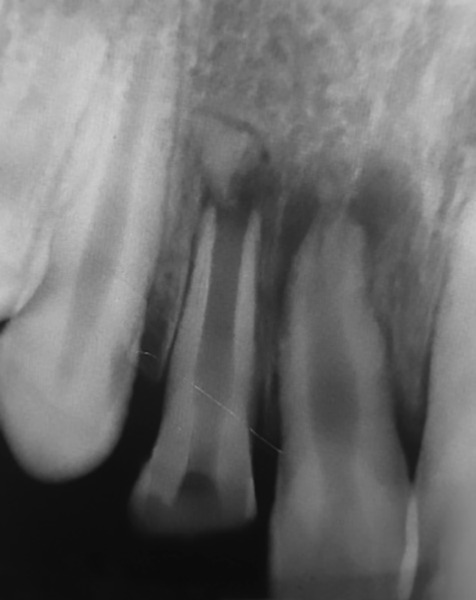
Intraoral periapical radiograph showing class III fracture with displaced apex and periapical radiolucency
